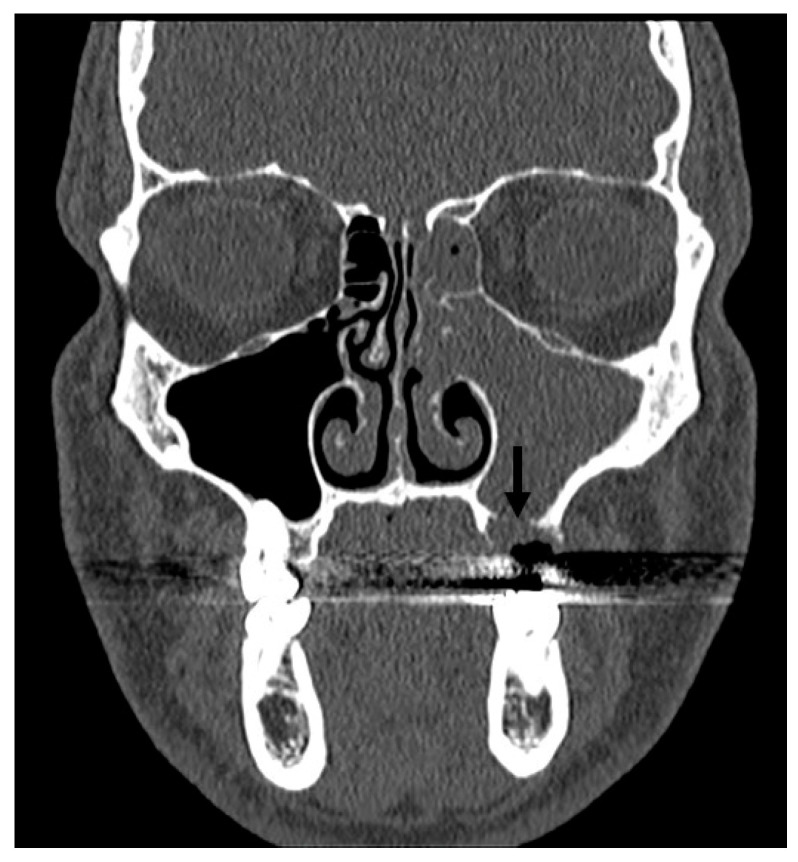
CT scan of odontogenic left maxillary sinusitis. OAF: oroantral fistula (arrow).
